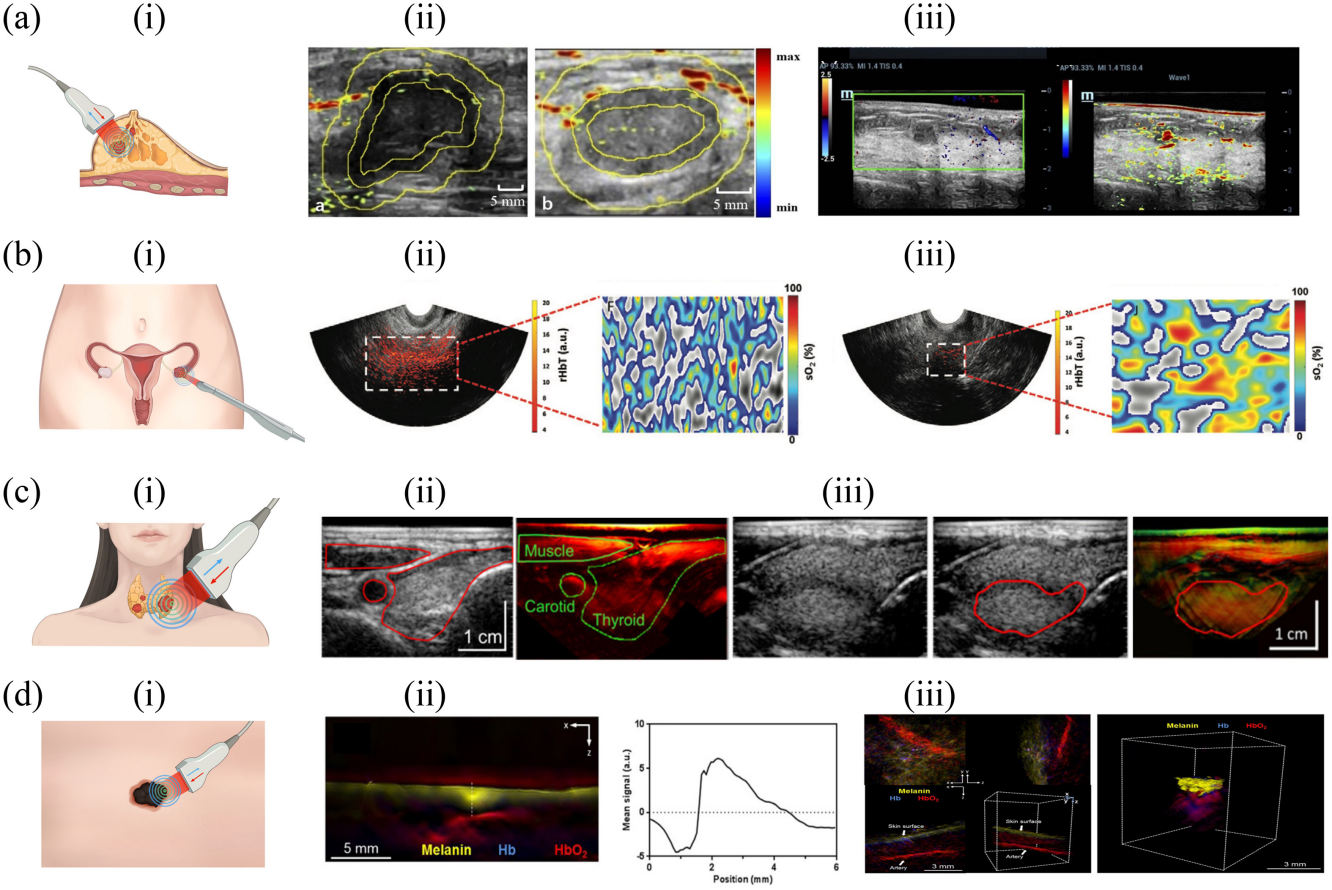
Representative *in vivo* PA imaging for tumors. (a) Breast tumor PA imaging: (i) a hand-held optoacoustic unit for breast tumor PA imaging. (ii) Left: PA/US modality imaging of a malignant breast lesion (F, 40 years), with limited PA signals in the central region and abundant signals in the peripheral and surrounding regions. Right: PA/US imaging of a benign breast lesion (F, 36 years) with abundant PA signals in all depicted regions. (iii) Intraductal papilloma (F, 65 years). Left: grayscale US with no blood signal indicated by color doppler flow imaging in the lesion. Right: abundant vessel signals indicated by PA. (b) Ovarian tumor PA imaging: (i) A hand-held optoacoustic unit for ovarian tumor PA imaging. Images of (ii) a 4.5 cm endometrioid adenocarcinoma (F, 63 years) and (iii) a 2.2 cm benign tumor (F, 63y). (ii) A coregistered US/PA image with extensive diffused vascular distribution (rHbT map) and an average ${\mathrm{SO}}_{2}$ in the region of interest (ROI) of 43.2% (${\mathrm{SO}}_{2}$ map). (iii) The scattered vascular distribution (rHbT map) and an average ${\mathrm{SO}}_{2}$ in the ROI of 58.2% (${\mathrm{SO}}_{2}$ map). (c) Thyroid nodule PA imaging: (i) A hand-held optoacoustic unit for thyroid nodule PA imaging. (ii) Thyroid MSOT imaging. Transversal US image (left) and MSOT image (right, showing HbT) of the thyroid gland and surrounding tissue. (iii) MSOT imaging of the benign thyroid nodule. (d) Skin cancer PA imaging: (i) A hand-held optoacoustic unit for skin cancer PA imaging. (ii) MSOT imaging of the superficial basal cell carcinoma acquired by the 2D probe and its distribution along the depth dimension. (iii) Left: 3D MSOT imaging of a viral wart, which identified the large artery by the characteristics of ${\mathrm{HbO}}_{2}$ absorption. Right: 3D MSOT imaging of a representative basal cell carcinoma lesion. Melanin signals (yellow) were clustered at the top with strong hemoglobin signals underneath the carcinoma (Hb: blue, ${\mathrm{HbO}}_{2}$: red). Panel a-ii) is reprinted with permission from Ref. 40 © The Optical Society. Panel a-iii is reprinted with permission from Ref. 41 © The Optical Society. Panels b-ii and b-iii are reprinted with permission from Ref. 42 © RSNA. Panels c-ii and c-iii are reprinted with permission from Ref. 43, originally published in JNM, © SNMMI. Panels d-ii and d-iii are reprinted with permission from Ref. 44, under the terms of Creative Commons CC BY license.
